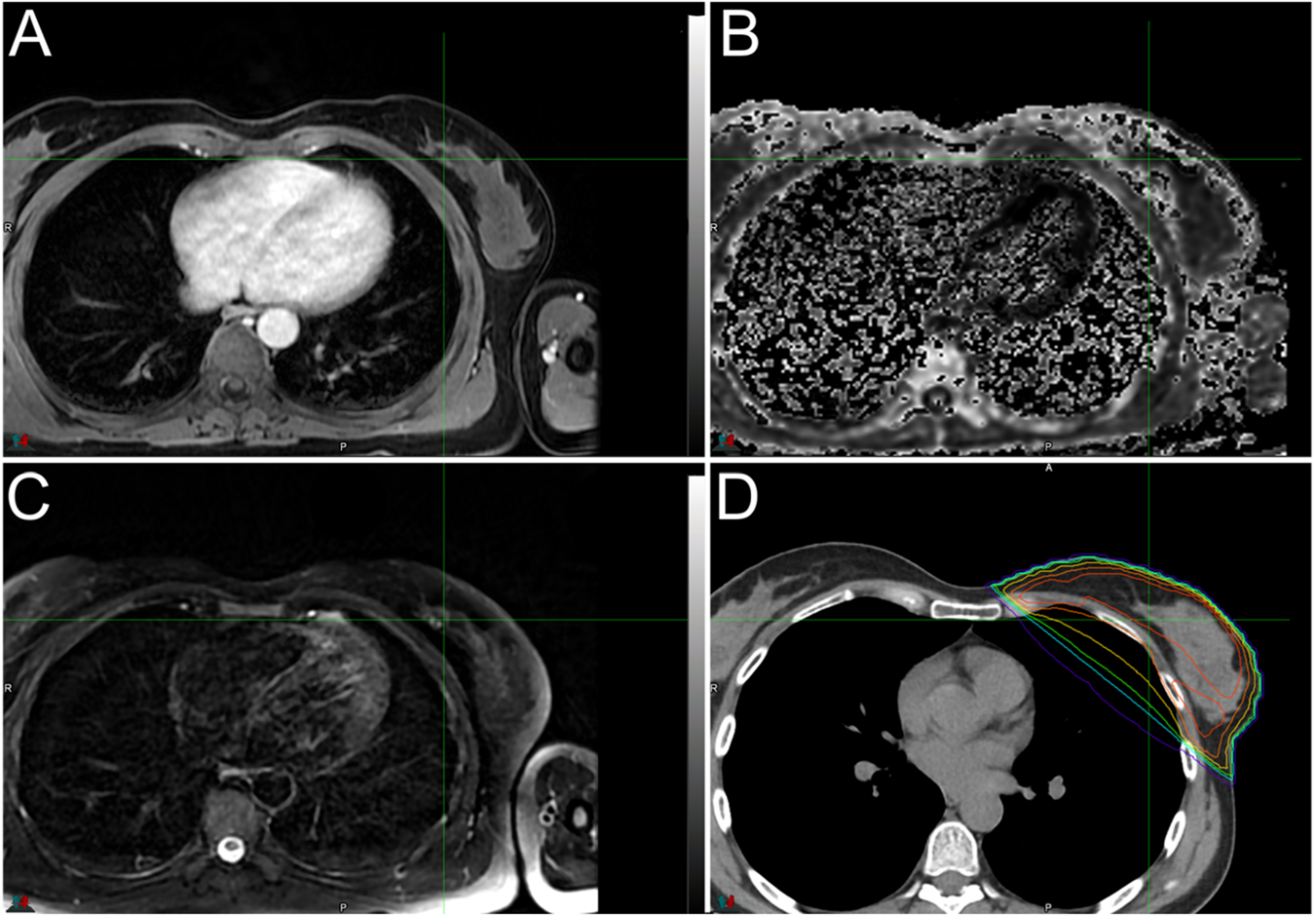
Contrast-enhanced fat-saturated T1w image (A), exponential ADC map (B), fat-suppressed T2w sequence (C), and related centering CT (D) with superimposed distribution of doses in a patient with breast cancer
